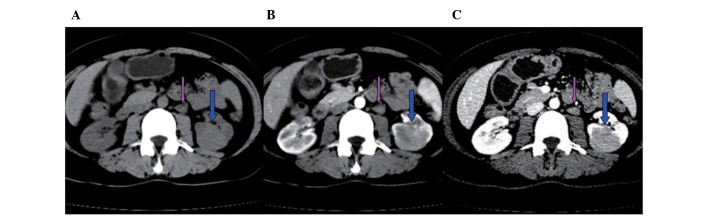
CT scans reveal that the mass (blue arrow) showed enhancement, was located in the lower pole of the left kidney and measured 38×30 mm. Metastasis was detected in an enlarged (20 mm) para-aortic lymph node (pink arrow). CT values; (A) plain scan, 40–60; (B) arterial phase, 71–89; (C) venous phase, 90–120. CT, computed tomography.
